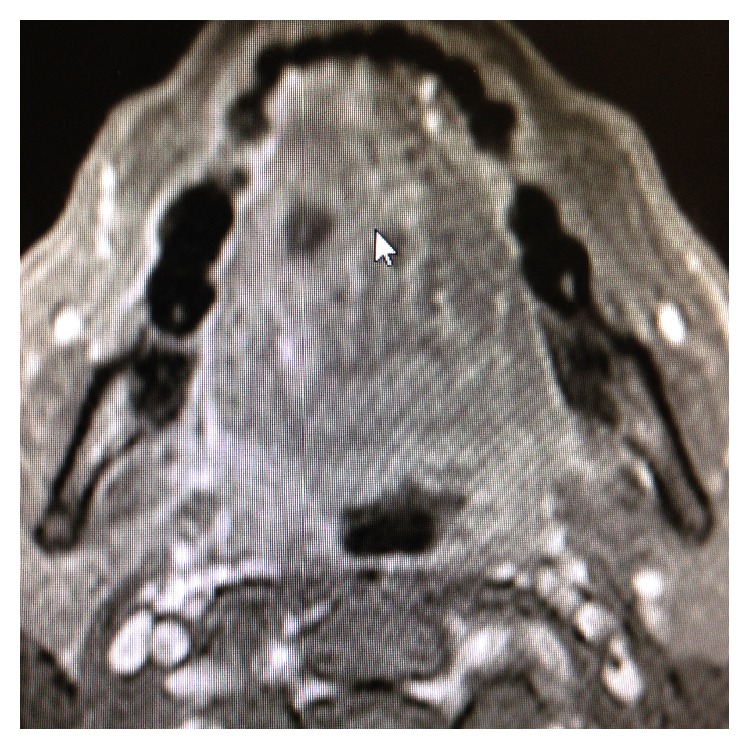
MRI of the neck revealing an anterior tongue mass.
